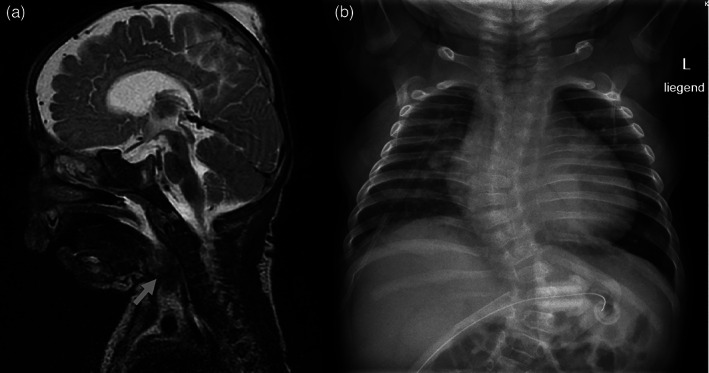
(a) Magnetic resonance imaging of the brain revealed intracranially wide inner and outer cerebrospinal fluid spaces and a very narrow corpus callosum. Note the increased fatty tissue in the epipharynx (grey arrow). (b) X‐ray of the chest showing cardiomegaly, scoliosis and paravertebral left residual consolidation with positive air bronchogram
